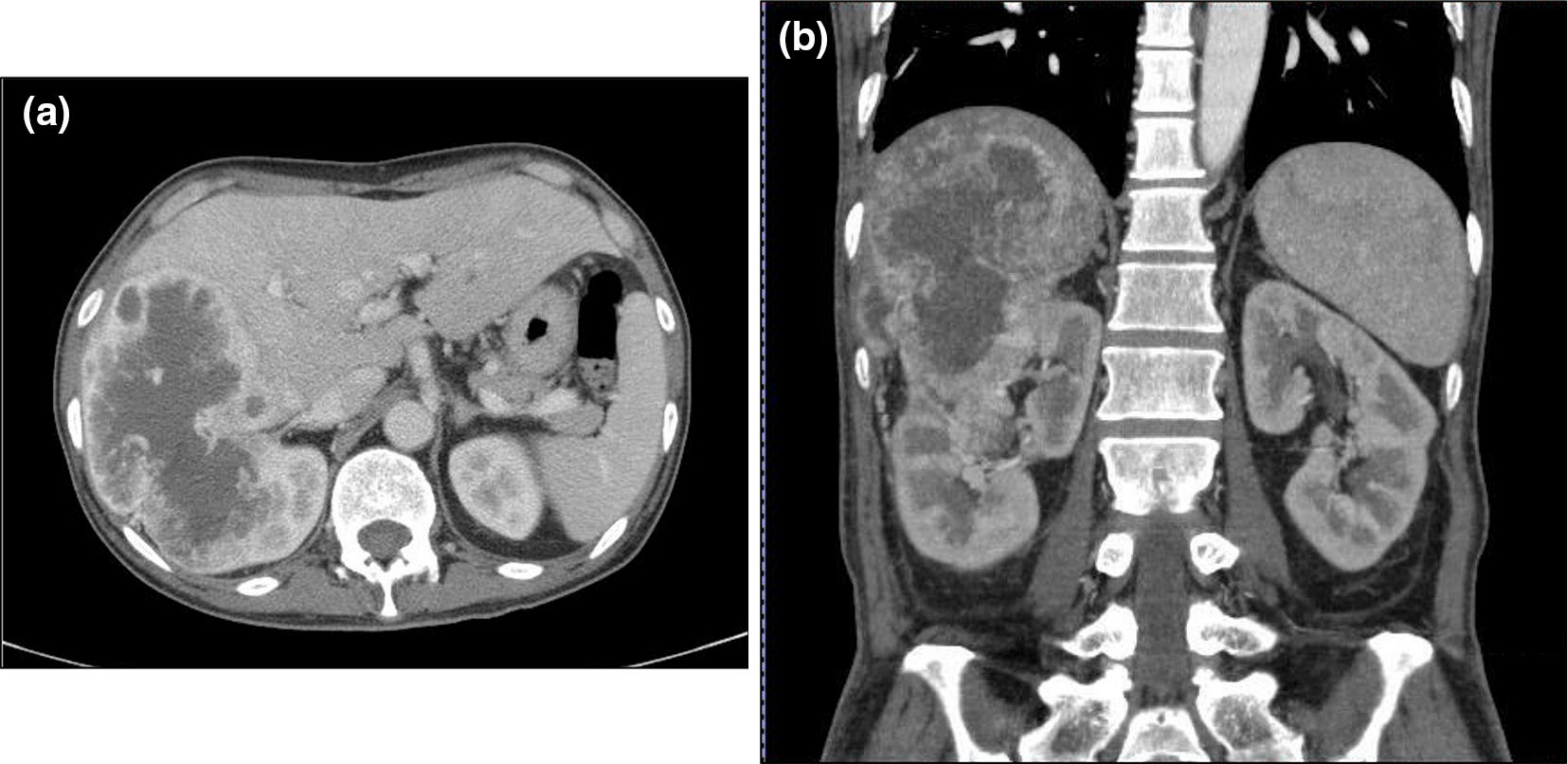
a A transverse and b a coronal computed tomography image. Preoperative computed tomography showed that there was a huge renal cell carcinoma (13 cm in diameter), invading the right lobe of the liver
